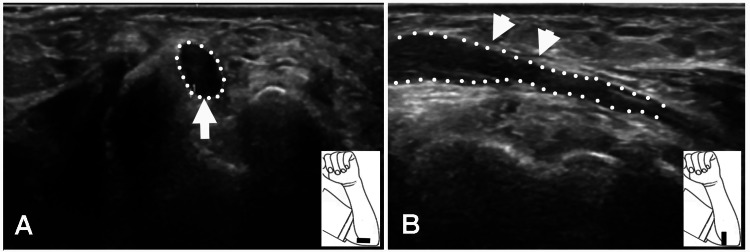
Ulnar Nerve on Ultrasound Studies Case 2: (A) Large hypoechoic ulnar nerve at the elbow (arrow). The arrow indicates the large diameter of the ulnar nerve. (B) A decrease in the diameter of the ulnar nerve in the cubital tunnel. The two arrows show the decreased diameter of the ulnar nerve.
